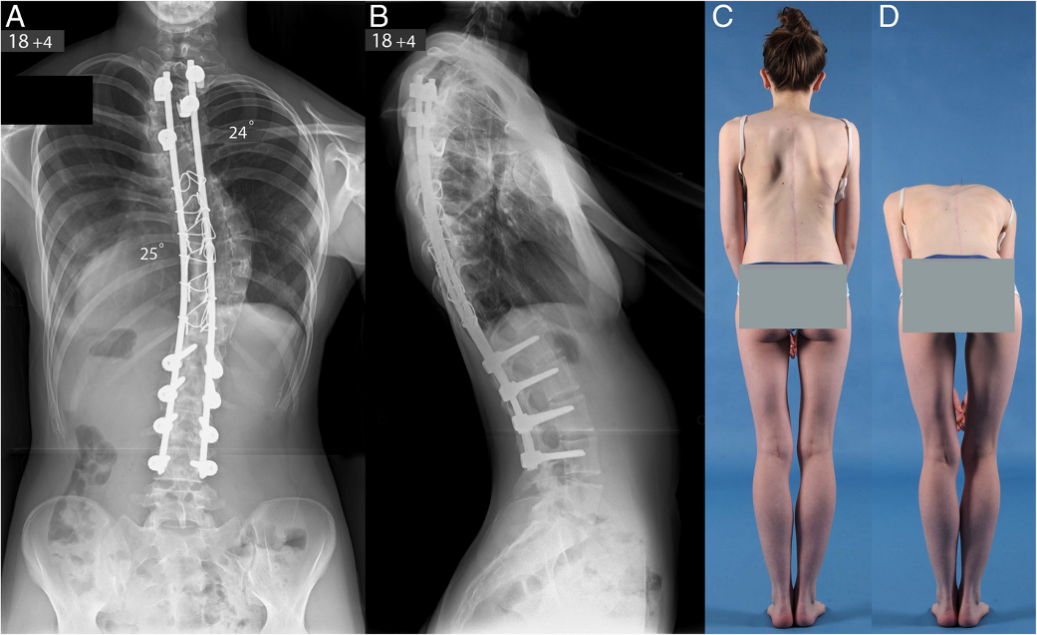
Posteroanterior (A) and lateral (B) radiographs of the spine and clinical photographs (C, D) at latest follow-up show excellent scoliosis correction across both thoracic curves and an overall balanced spine in the coronal and sagittal planes.
